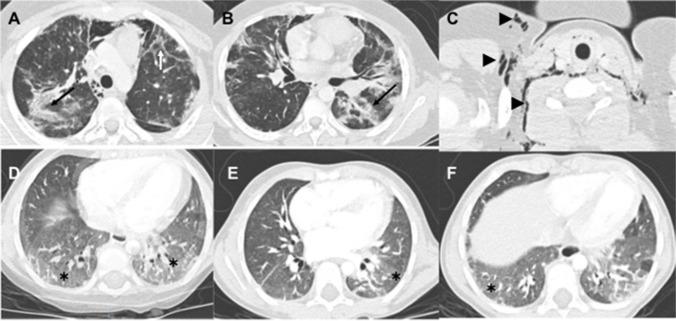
High-resolution computed tomography (HRCT) findings in two pediatric patients with anti-MDA5-positive juvenile dermatomyositis and interstitial lung disease. Patient 1: A–B Axial HRCT images from patient 1 demonstrates alveolar ground glass consolidation (black arrow) with smooth interlobular septal thickening (white arrow). C Axial HRCT demonstrates a pneumomediastinum (arrowhead) in the absence of pneumothorax, a known complication in severe ILD associated with anti-MDA5 autoantibodies. Patient 2: D–F Axial HRCT images from patient 2 demonstrates bilateral diffuse ground-glass opacification (asterisk)with areas of relative subpleural sparing.
